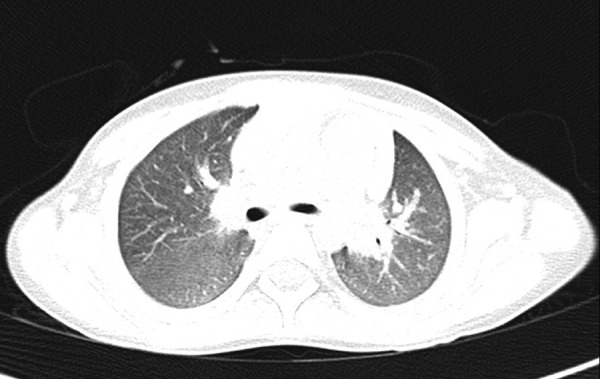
Computed tomography scan of chest and abdomen show hepatosplenomegaly and diffuse ground glass haze in both lung fields.
